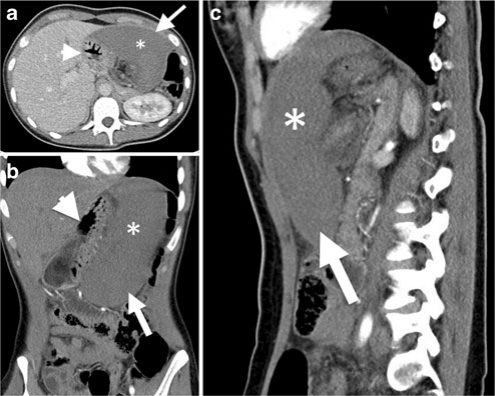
Splenic torsion. (a) Axial, (b) coronal and (c) sagittal contrast-enhanced CT images of a 17-year-old female with a history of recurrent abdominal pain and suspected Familial Mediterranean Fever show anteromedially displaced spleen (*arrows*). No contrast enhancement was observed in spleen parenchyma (*asterisks*), suggesting splenic infarct secondary to splenic torsion. Stomach (*arrowheads*) is posteromedially compressed by enlarged and torsed spleen
